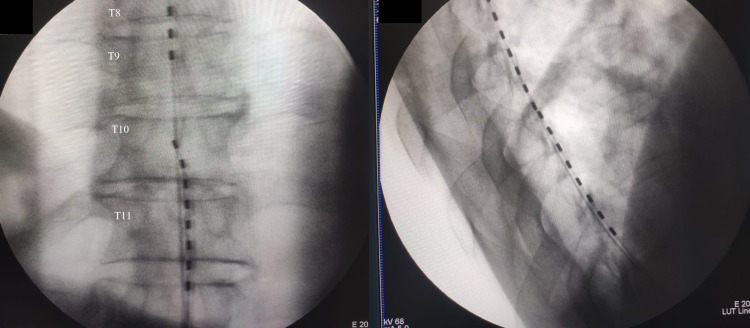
Intraoperative X-ray during spinal electrode placement.
